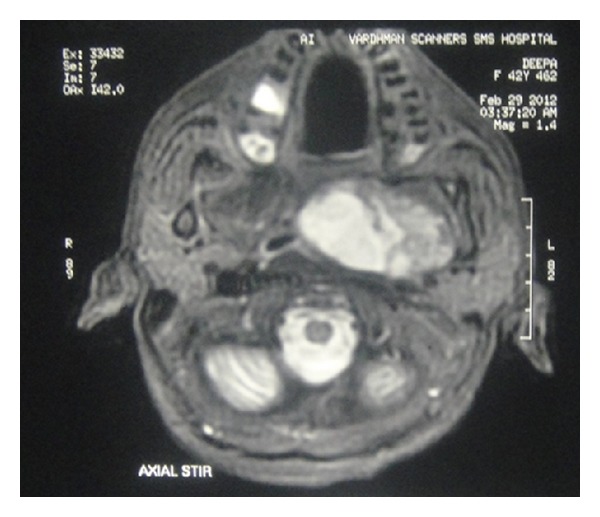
T1-weighted post-Gd MRI image showing preservation of a fat plane between the tumor and parotid gland.
